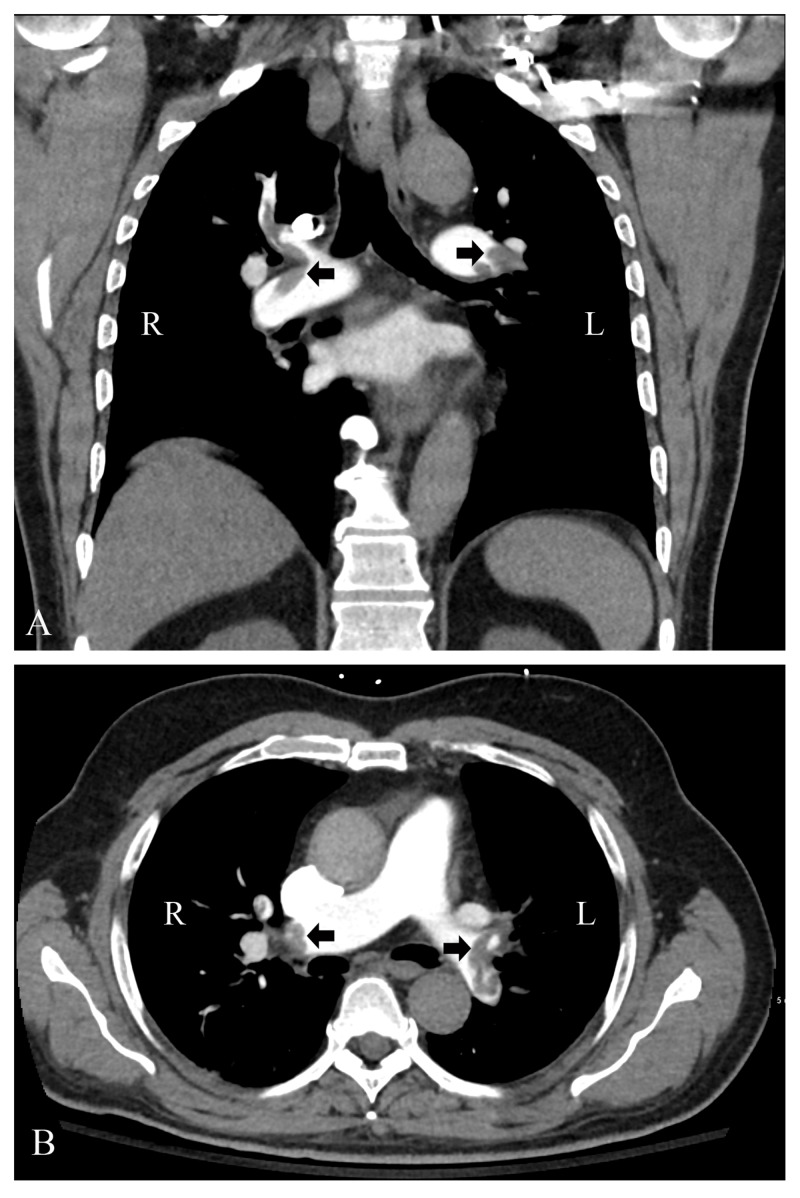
CT angiogram of chest. (A) Coronal view and (B) axial view showing bilateral filling defects representing pulmonary embolism. R, right lung; L, left lung.
